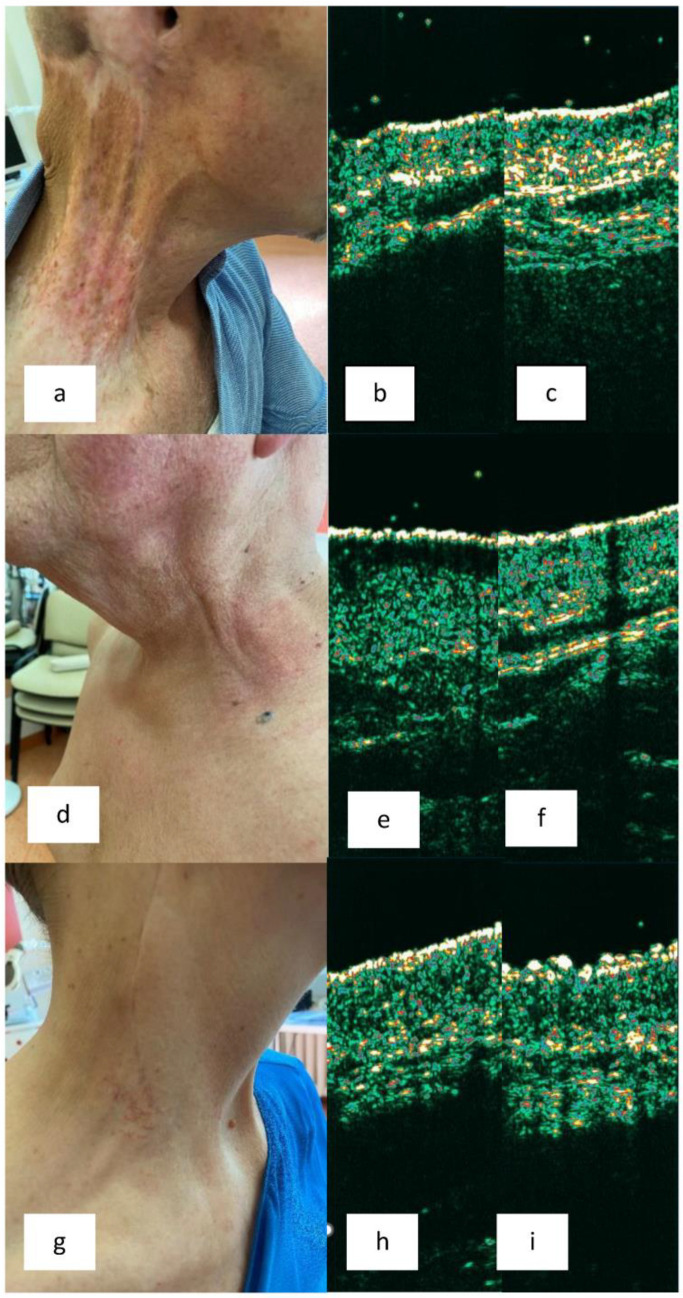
Clinical presentations and their corresponding skin sonograms of the irradiated and control areas. (a) Patient with BCC of the external ear with lymph node metastases. 2 years post unilateral radiotherapy (RT). Grade 3 of chronic radiation induced skin injury (cRISI): atrophy, erythema, discoloration, limited mobility. (b,c) Sonogram of irradiated skin (b) and contralateral non-irradiated skin (c). Skin thinning is visible. (d) Patient with tonsil cancer with lymph node metastases. 5 years post bilateral RT. Grade 2 cRISI: erythema and swelling. (e,f) Sonogram of irradiated skin (e) and surrounding non-irradiated skin (f). Subepidermal low echogenic band (SLEB) is visible. (g) Patient with melanoma in the temporoparietal region with lymph node metastases. 6 years post unilateral RT. Grade 1 cRISI: slight atrophy, telangiectasia, subjective symptoms. (h,i) Sonogram of irradiated skin (h) and contralateral non-irradiated skin (i). Flattened entry echo and slight skin thinning are visible.
